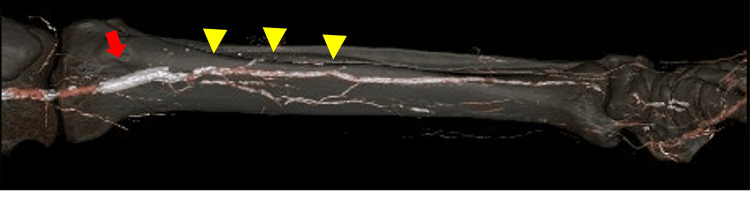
Contrast-enhanced computed tomography (CECT) image Both the anterior tibial artery (ATA) and the posterior tibial artery (PTA) were jailed by the SMART stent (red arrow) and the peroneal artery (yellow arrowhead) could be only detected.
